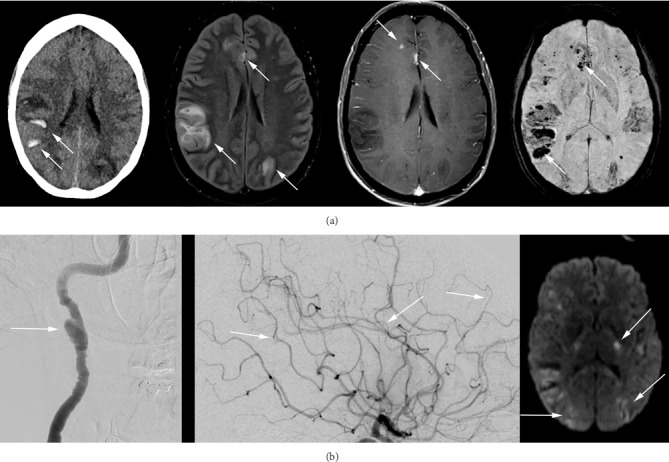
(a) Left to right: Repeat CT, FLAIR, T1post, and SWI showing marked interval progression of hemorrhages on CT and SWI with surrounding edema on FLAIR and subtle foci of enhancement on T1 post-contrast images (arrows). The vasogenic edema and moderate-sized right temporoparietal hemorrhage caused cerebral edema and midline shift, and there were multiple additional petechial hemorrhages. There was also new sulcal FLAIR signal with subtle enhancement consistent with blood-brain barrier breakdown. (b) Left to right: Repeat catheter angiography showed a new right cervical internal carotid artery dissection on carotid angiography (arrow), progression of luminal irregularities in the distal the middle cerebral artery and the anterior cerebral artery branches (arrows), and new areas of cytotoxic edema/infarction on DWI (arrows).
